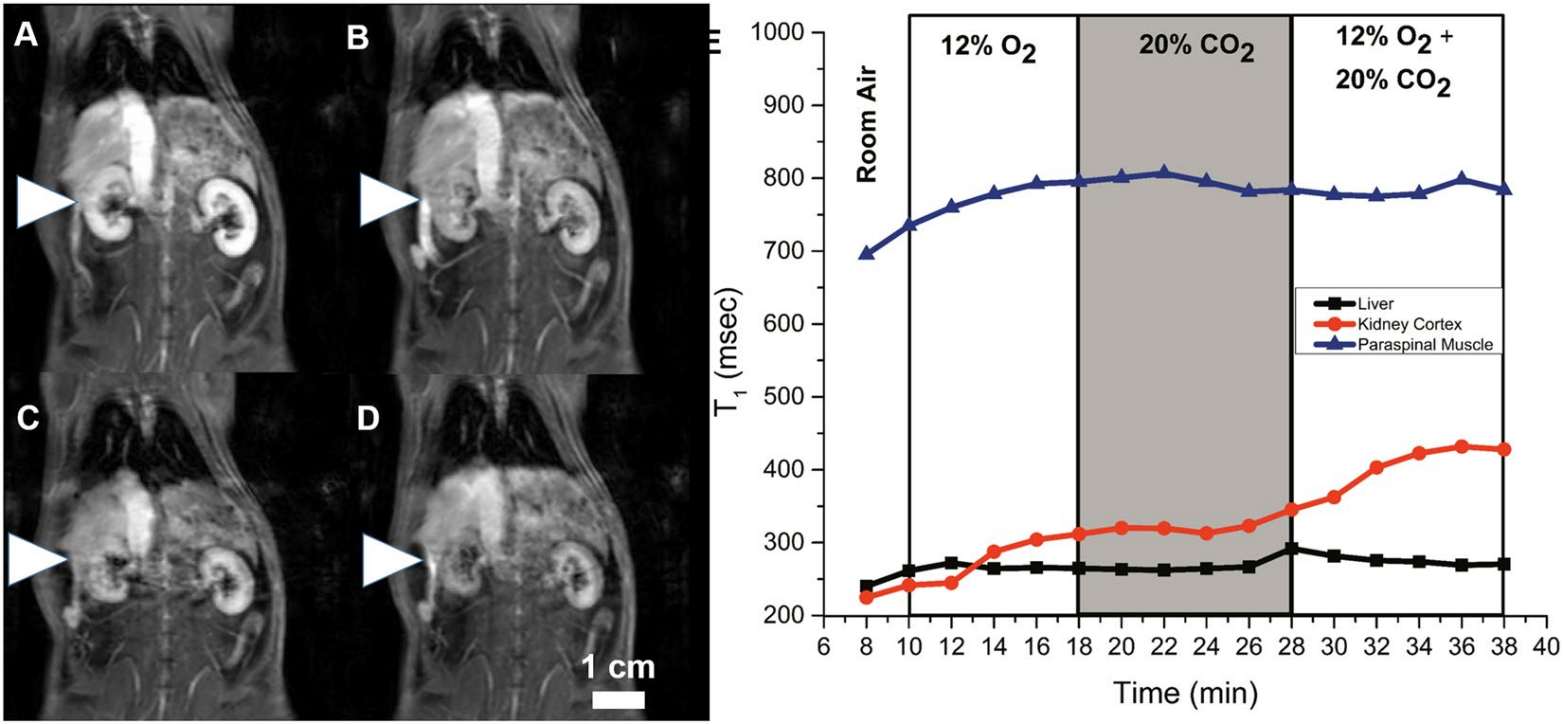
Dynamic MR imaging of differential microvascular response to hypercapnia and hypoxia. The kidney is shown on *T*
